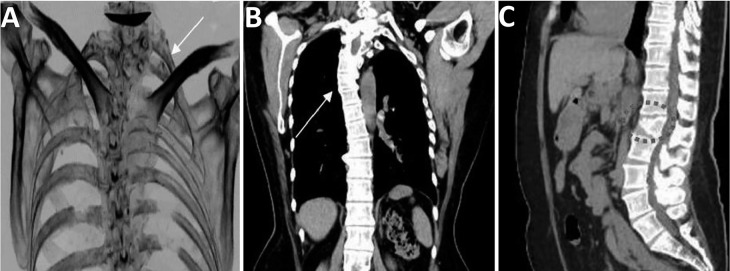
Bone assessment. (A) CT bone window, left supernumerary rib in the seventh cervical vertebra (B) CT coronal slice, high dorsal scoliosis with right concavity vertebral fusion in the c5/c6 vertebrae (C) CT sagittal slice, vertebra with butterfly appearance in the lumbar region (L2).
